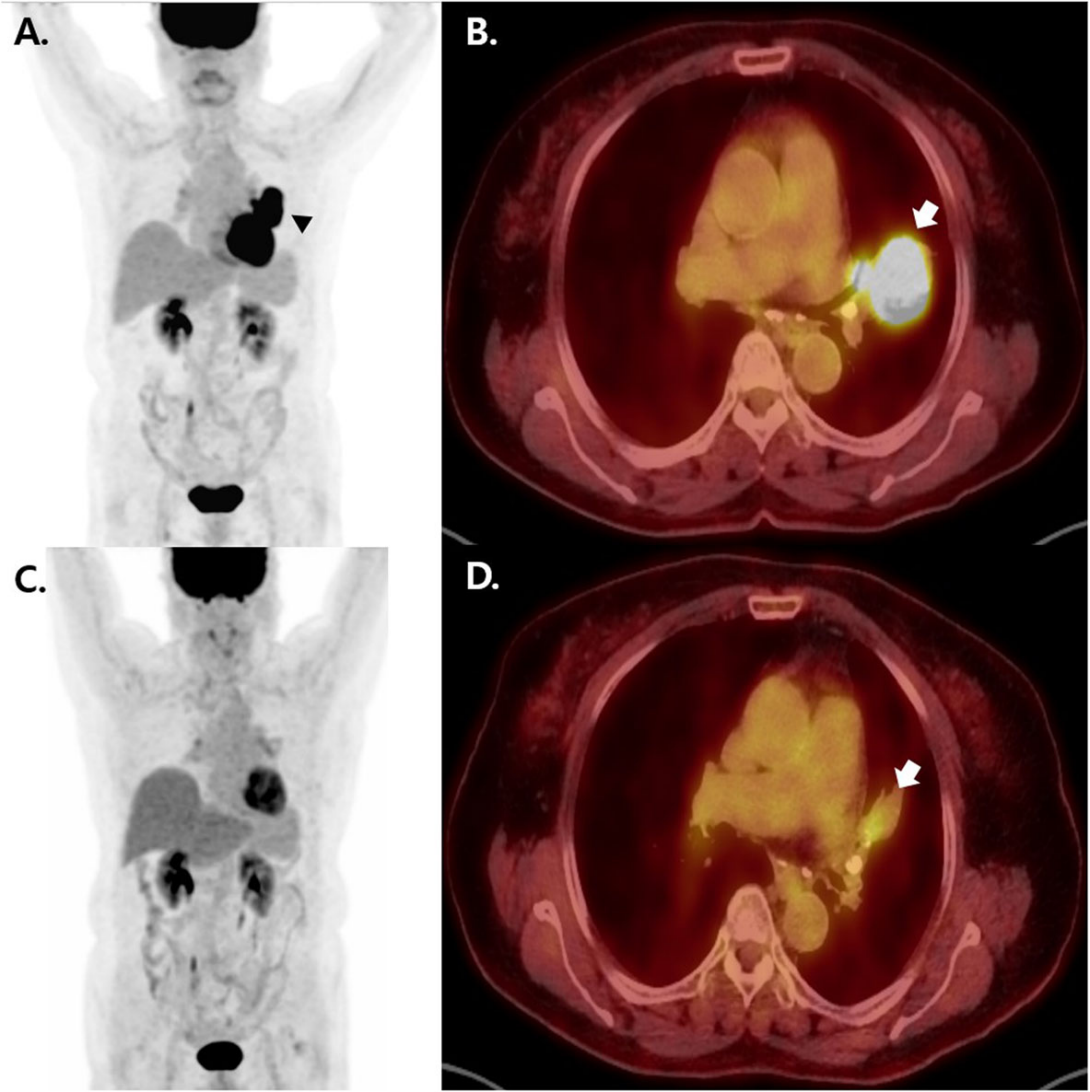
Comparison of in ^18^F-fluorodeoxyglucose positron emission tomography/computed tomography images before and after spontaneous remission of the left lung mass. a and b: A coronal positron emission tomography (PET) image in January 2015 revealed a mass with intense uptake (arrowhead) in the left lung (a) corresponding to a soft tissue density in a combined PET and CT fusion image (arrow). c and d: After the spontaneous remission, decreases in both size and uptake of the left lung mass were identified on a coronal PET image (c) and a combined PET and CT fusion image (arrow) (d) in October 2018
